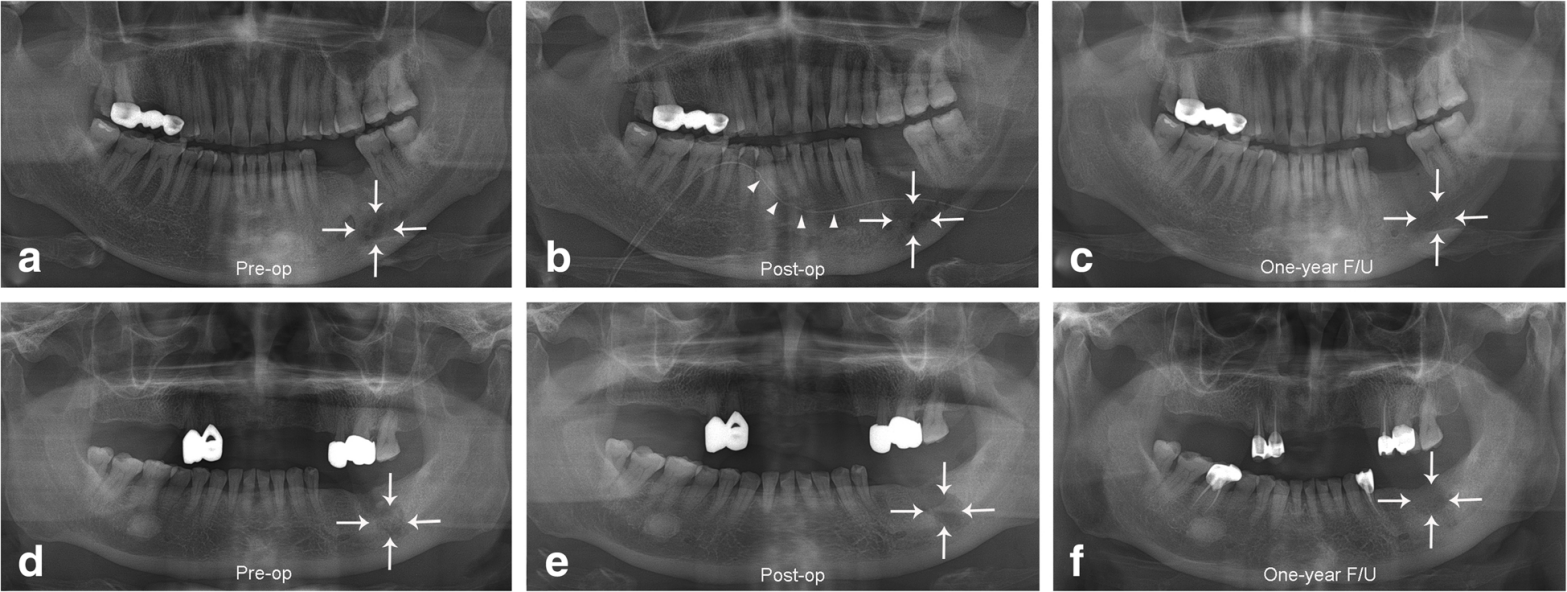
A panoramic radiograph showing the comparison of sclerosing type OM with and without drainage.. Pre-operative sclerosing OM on panoramic X-ray shows as a dense lesion with increased radiopacity compared to the surrounding healthy bone tissue (marked with arrows) in patient No. 22 (a). Immediate post-operative view following saucerization and drain insertion for decompression (marked with arrowheads). Panoramic X-ray view may show only the lingual wall of mandibular body with minimum soft tissue image. b. Following the decortication procedure, the sclerosing OM could be healed uneventfully with bone turnover, resulting in the decrease of bone density in the sclerotic area (the decrease of GSV) with minimal difference from the surrounding healthy bone tissue at one-year follow-up (c). Pre-operative panoramic radiograph showing sclerotic OM in the left posterior mandible region in patient No.67 (d). Immediate post-operative view without drain (e). One-year follow-up view (f)
